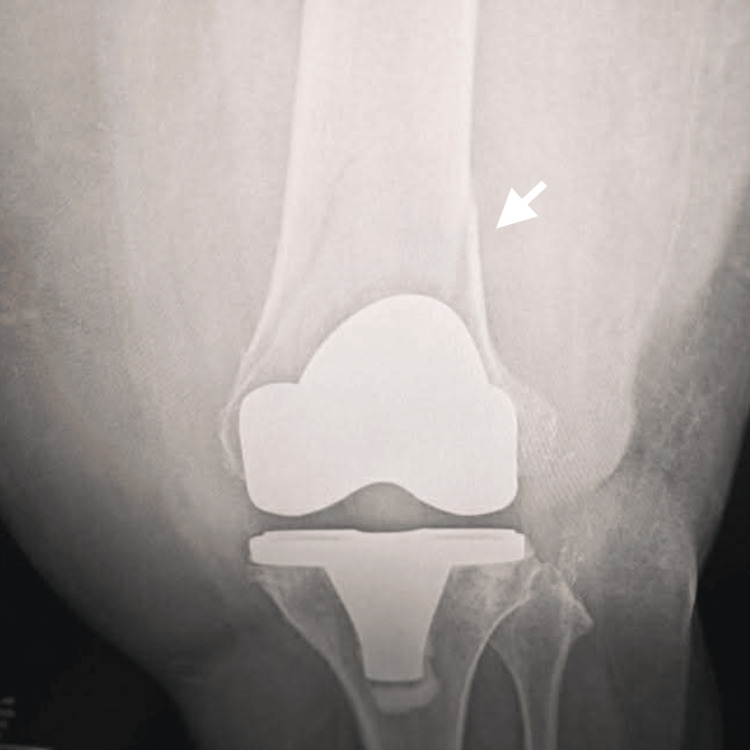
Anteroposterior radiograph of the left knee Anteroposterior radiograph of the left knee showing a displaced supracondylar periprosthetic distal femoral fracture adjacent to the femoral component of the total knee arthroplasty. The arrow highlights the fracture site above the prosthetic component.
